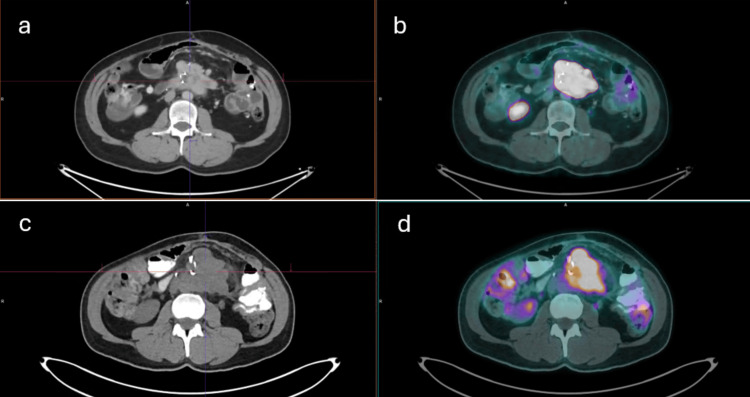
(a, b) Axial CT and axial SSR PET/CT images obtained in December 2024 demonstrating a radiotracer-avid nodal conglomerate in the mesenteric root, consistent with metastatic neuroendocrine disease (SUVmax 70.6). (c, d) Corresponding axial CT and axial ¹⁸F-FDG-PET/CT images obtained in May 2025 demonstrating increased ¹⁸F-FDG uptake within the same mesenteric mass (SUVmax 9.8), reflecting heterogeneous metabolic activity PET/CT: positron emission tomography/computed tomography, ¹⁸F-FDG: ¹⁸F-fluorodeoxyglucose, SSR: somatostatin receptor
